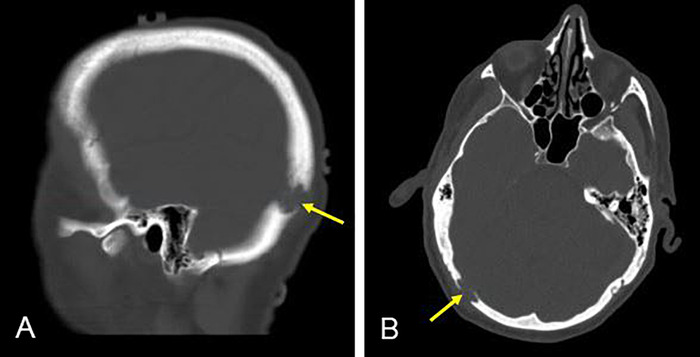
Computed tomography head with contrast. Sagittal (left) and axial (right) views demonstrate a 1.4-cm focal bony erosive lesion in the right occipital calvarium with ill-defined margins. Deformity and defect extend through both tables of the calvarium.
